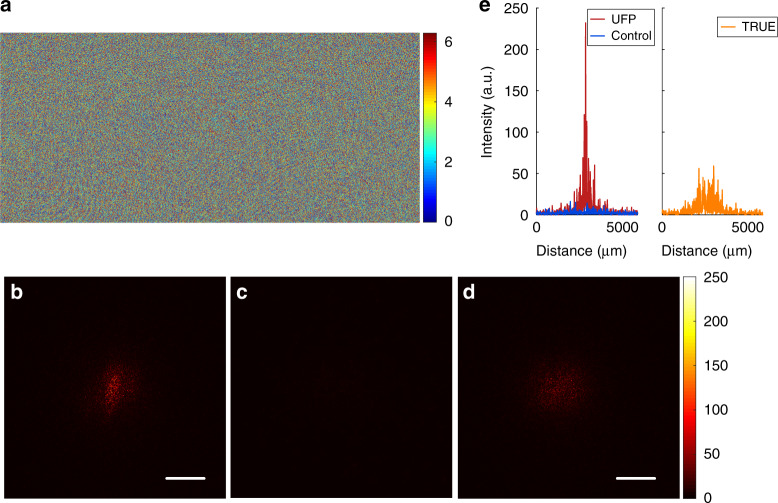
UFP optical focusing into scattering media via a full-phase modulation scheme. a Phase map displayed on the SLM, based on the differential signal between the measured complex fields when the ultrasound was OFF and ON. b Time-reversed focus from UFP optical focusing. c No optical focus was observed in a control experiment conducted without launching the ultrasound. d Time-reversed focus from conventional TRUE optical focusing under the same condition. e Line profiles of the central rows in b–d. Scale bars: 1 mm
